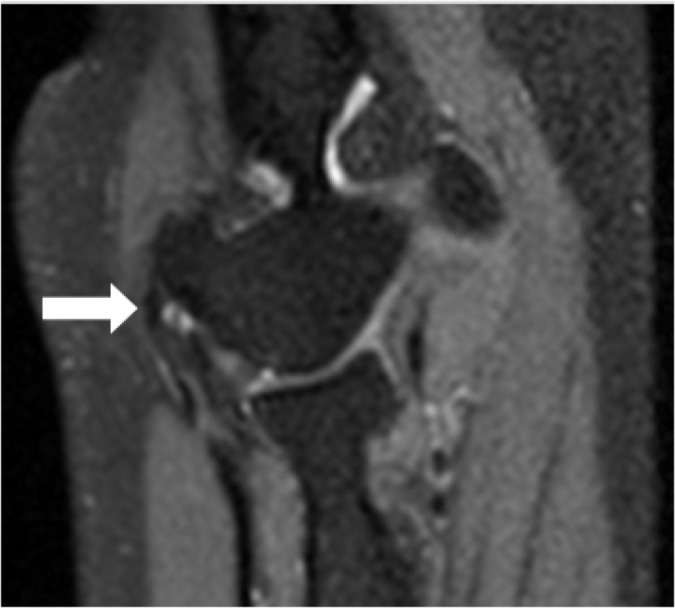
3D proton density fat saturated coronal magnetic resonance image of the left elbow showing fluid signal at the insertion of common extensor tendon on the lateral epicondyle, suggestive of enthesitis (arrow).
